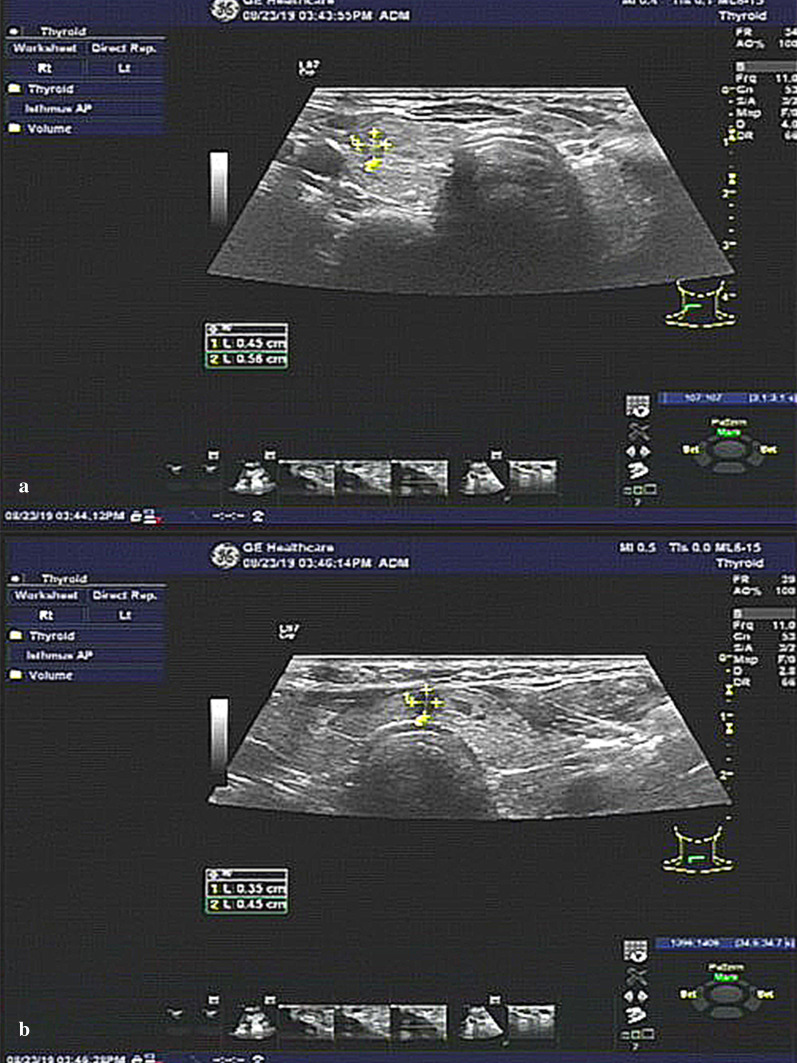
a Ultrasound imaging demonstrated a nodule (0.51 × 0.47 × 0.47 cm) that was located at the middle and upper part of the right thyroid gland; b Ultrasound imaging demonstrated a nodule (0.50 × 0.54 × 0.28 cm) that was located at the isthmus of the thyroid
